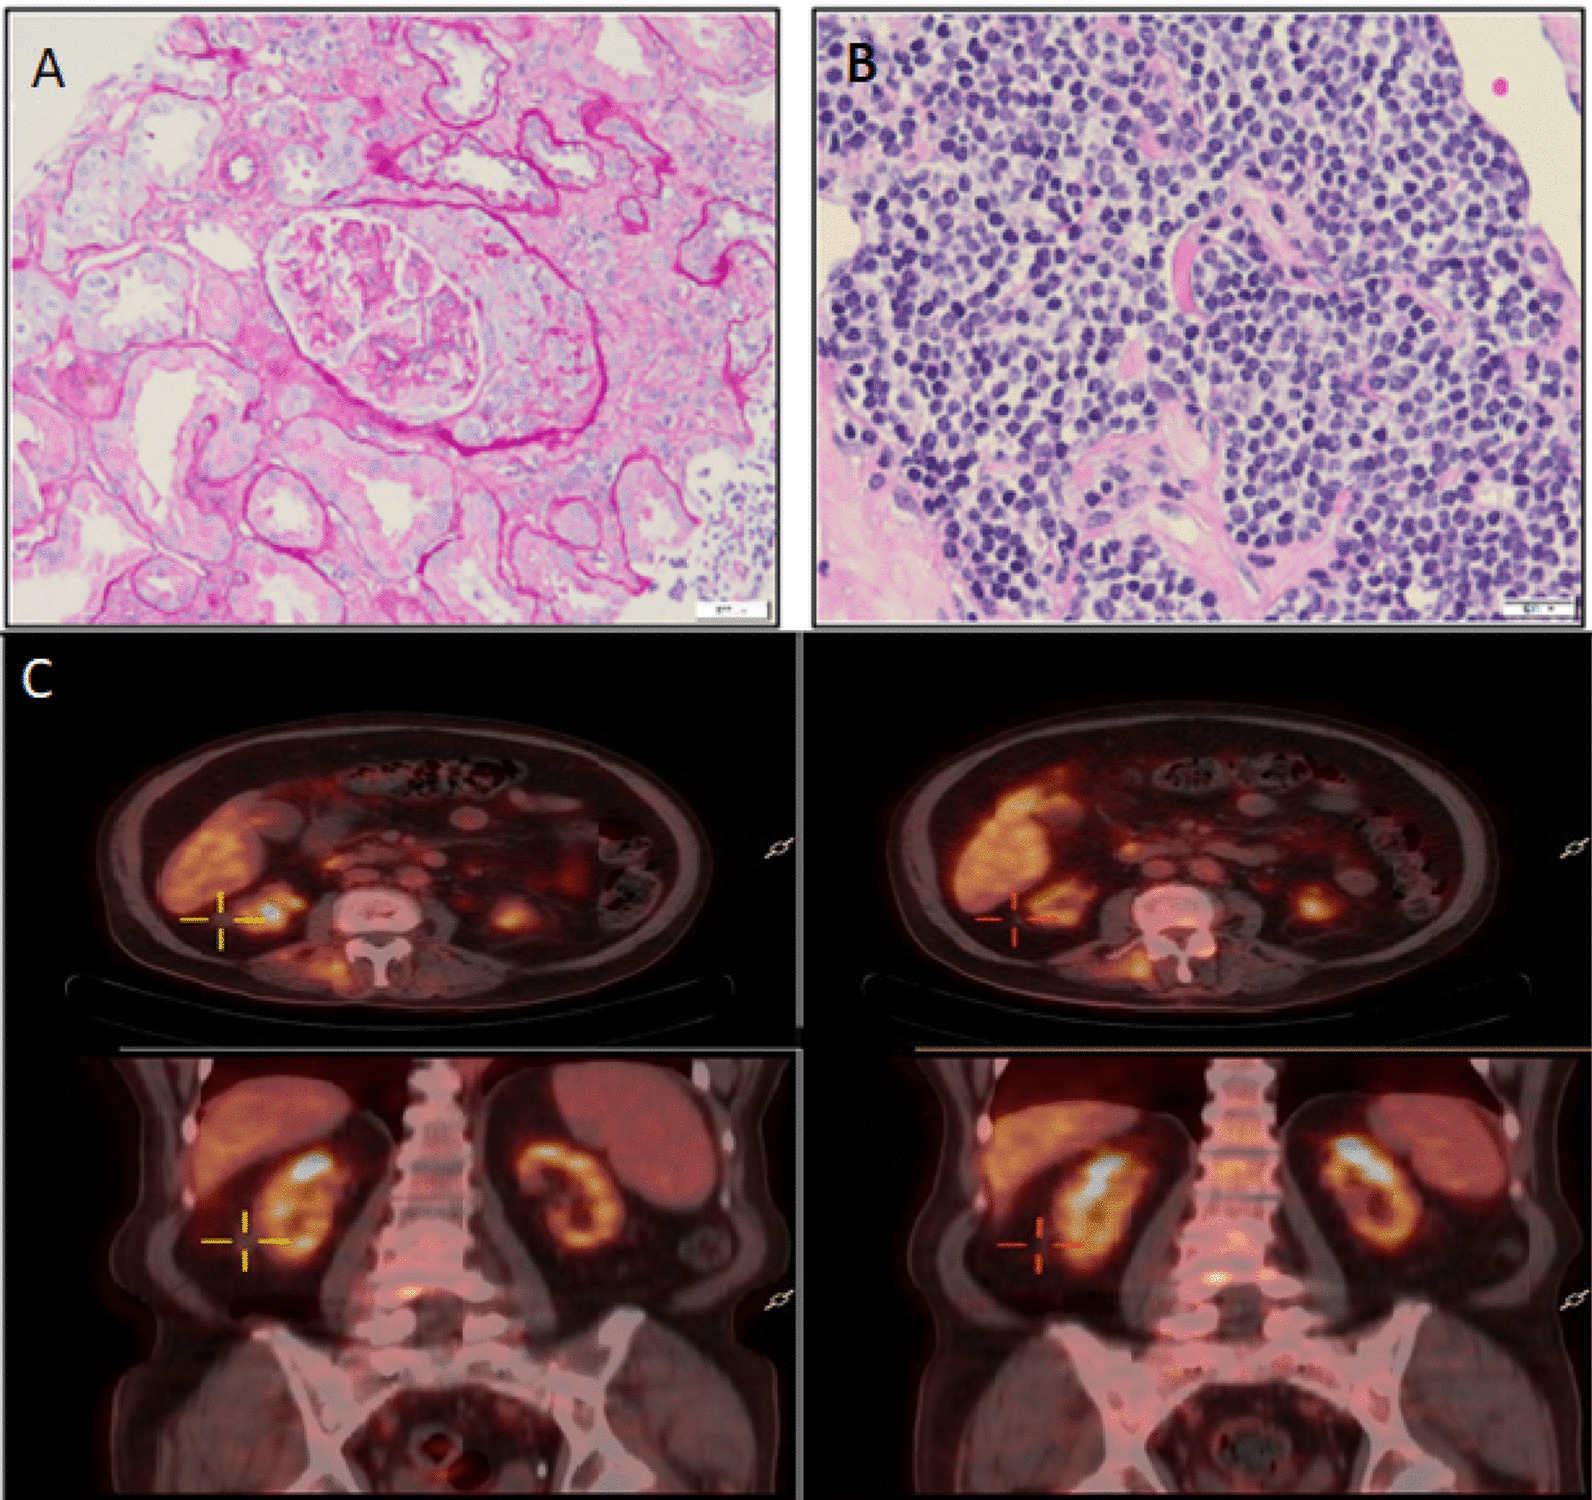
Kidney biopsy results: A Focal tubular atrophy and a glomerulus with cellular crescent are noted B The interstitial compartment shows, in addition to the inflammatory cell infiltrate, aggregates of monomorphic neoplastic lymphoid cells. PET-CT scan C on the left image showing a mass on the lower pole of the left kidney (yellow crosshair). Right image of PET-CT scan shows resolution of the mass after 3 months of chemotherapy
